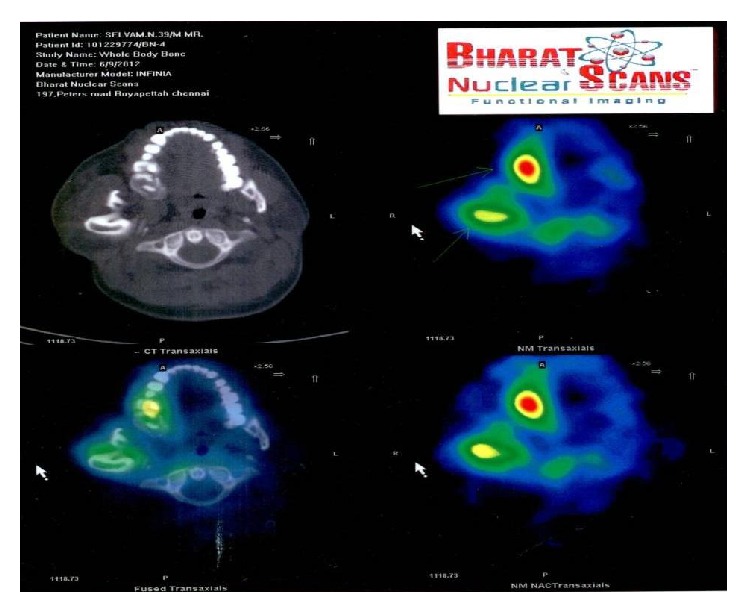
Bone scintigraphy shows increased uptake and hyperactivity in right sided maxilla and mandible of the face.
